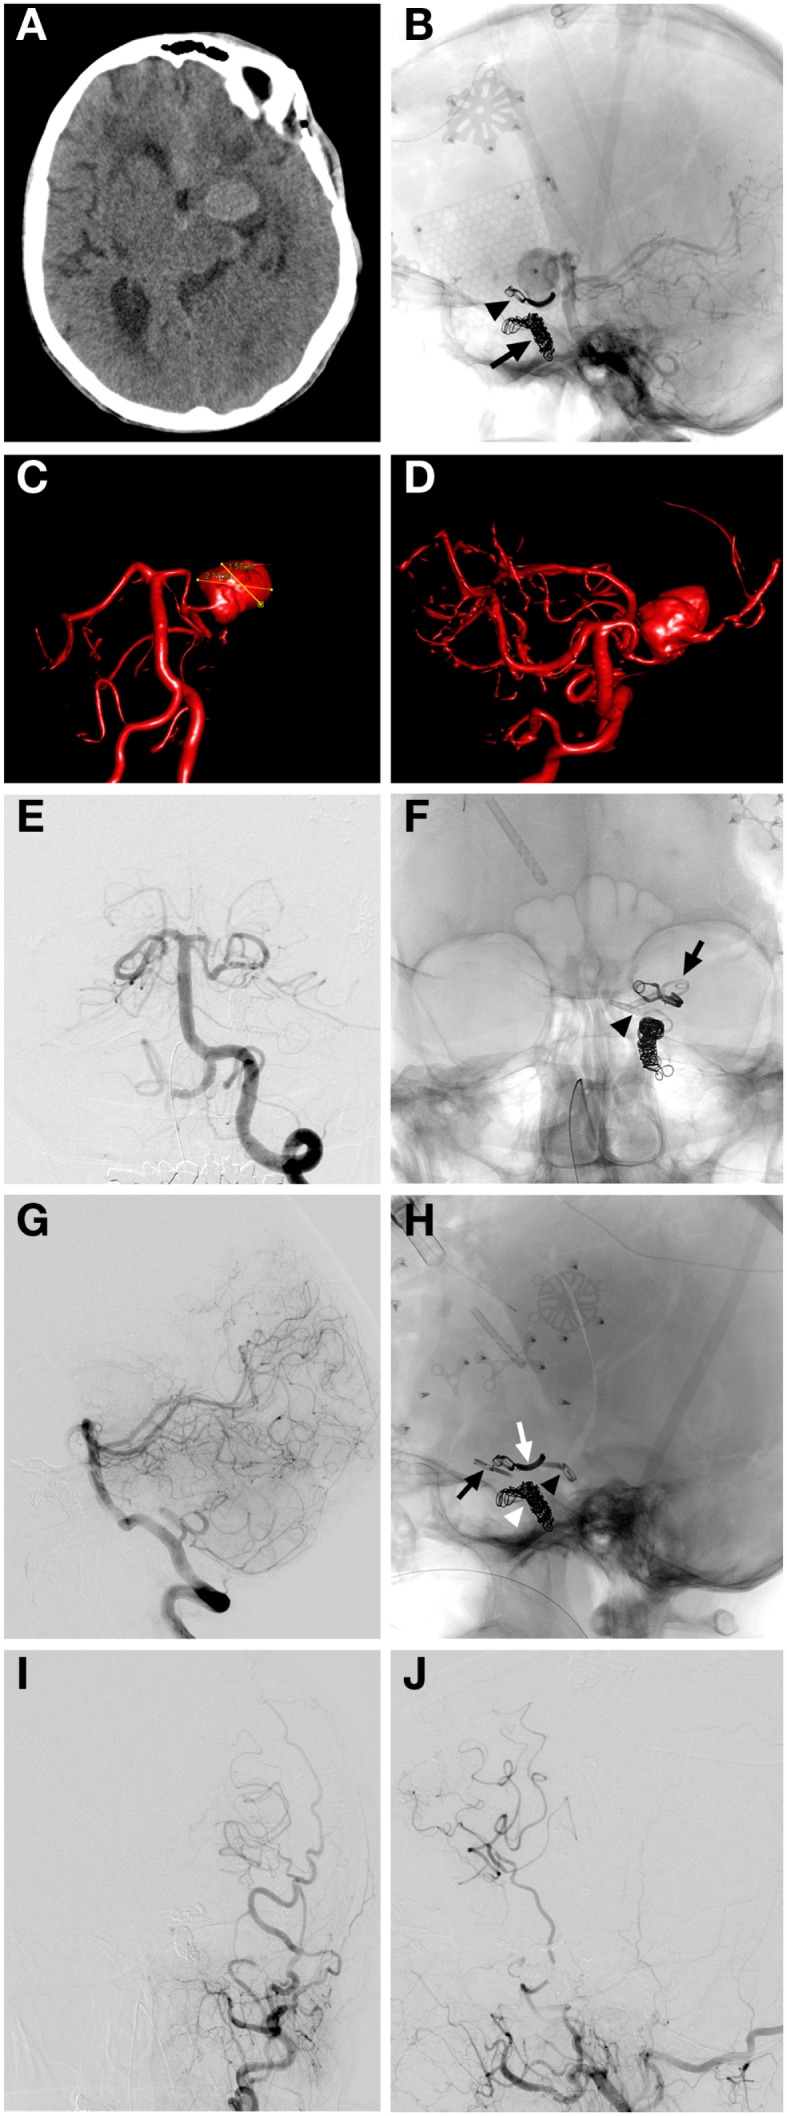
Case 2. (A) Non-contrast CT demonstrating a hyperattenuated left sylvian lesion with subarachnoid blood around the aneurysm and anterior interhemispheric fissure; (B) lateral unsubtracted skull radiograph showing the previously placed microsurgical clip (arrowhead) and coil mass from previous left ICA coil occlusion (arrow); (C,D) AP and oblique 3D angiographic reconstructions of vertebrobasilar system demonstrating the giant left supraclinoid ICA aneurysm being supplied by the left PComm; (E) AP subtracted angiographic view of left vertebral artery injection showing no filling of the giant aneurysm post clipping of left Pcomm; (F) AP skull x-ray demonstrating the two microsurgical clips used for feeding artery occlusions of a giant aneurysm with one clip over the Pcomm (arrowhead) and one over the supraclinoid ICA segment (arrow), previous coil mass from LICA occlusion and curved clip from previous clipping also seen; (G) lateral projection left vertebral artery angiographic injection showing no residual filling of giant aneurysm by the Pcomm; (H) lateral skull x-ray film demonstrating the clip from prior clipping (white arrow), coil mass from previous left ICA occlusion (white arrowhead), left Pcomm clip at the left Pcomm-P1 junction (black arrowhead), left supraclinoid ICA clip (black arrow); (I,J) AP and lateral left external carotid angiographic projections demonstrating filling of left superficial temporal artery with a patent bypass to left MCA and filling of left MCA territory post microsurgical occlusion of arterial feeders to aneurysm.
